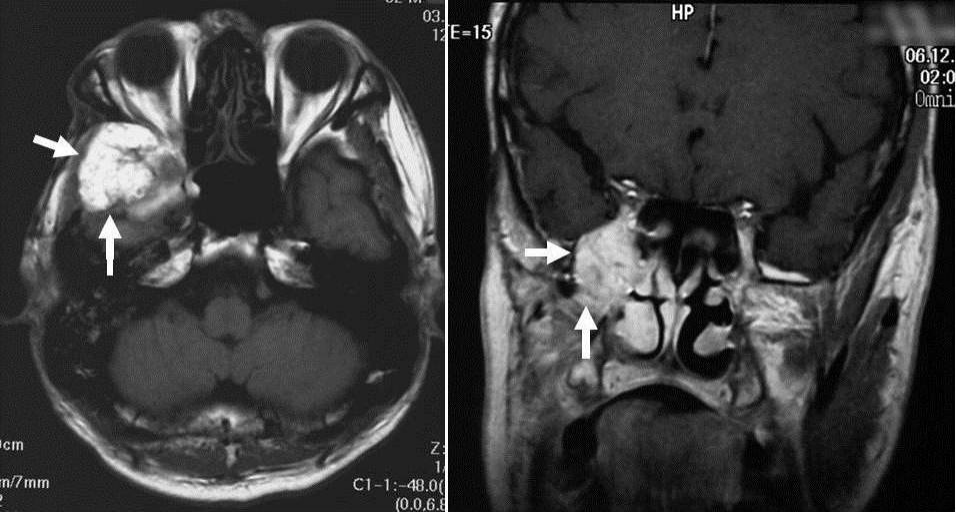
MRI findings (axial section and coronal section): The tumor (arrow) in the right temple showed low signal intensity on T1 weighted image (left) and enhanced by the contrast medium. (right).
